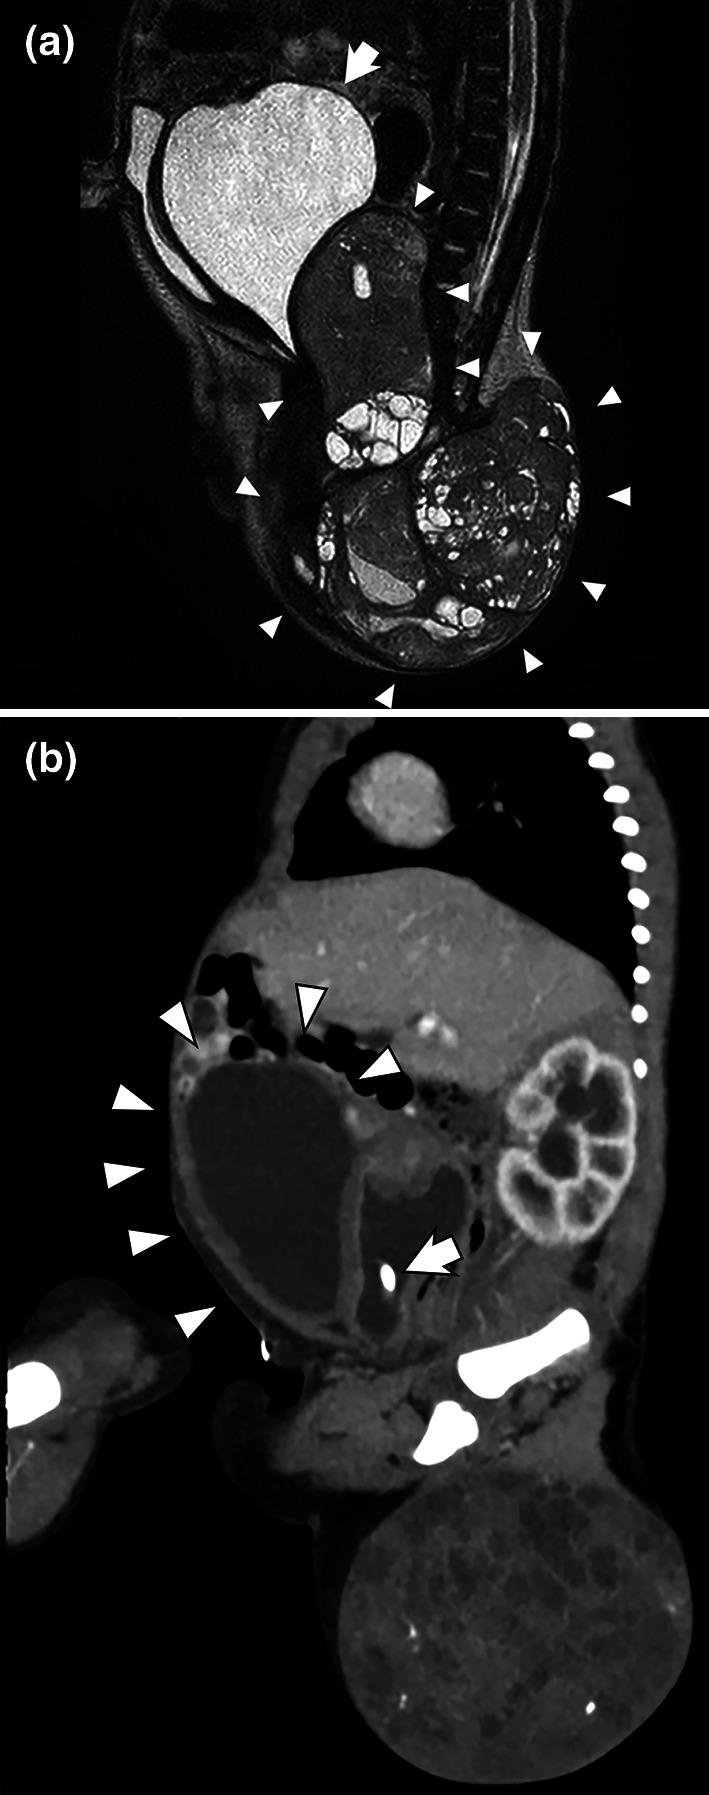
a Sagittal T2-weighted magnetic resonance imaging at 1 day of age through the pelvis shows an 11 cm mass indicating Altman’s type II sacrococcygeal teratoma (arrowhead) and hydrocolpos (arrow). b Computed tomography imaging at 2 days of age shows a shrunken hydrocolpos, an inversely dilated bladder (arrowhead), and bilateral grade 2 hydronephrosis. An indwelling catheter is inserted into the vaginal cavity (arrow)
